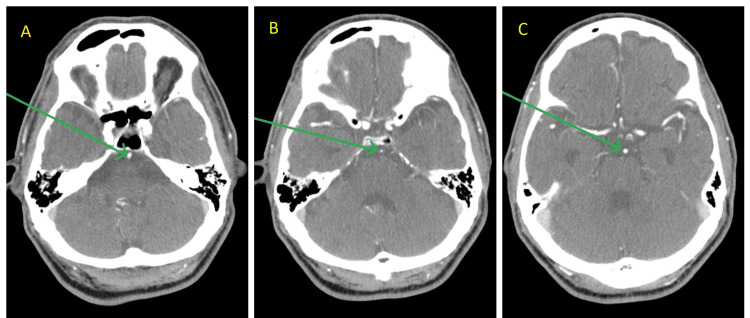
CT angiography head and neck Images (A)-(C) show short-segment severe stenosis of the basilar artery at three points (arrows), where maximum stenosis is apparent in Image (B). CT: computed tomography
